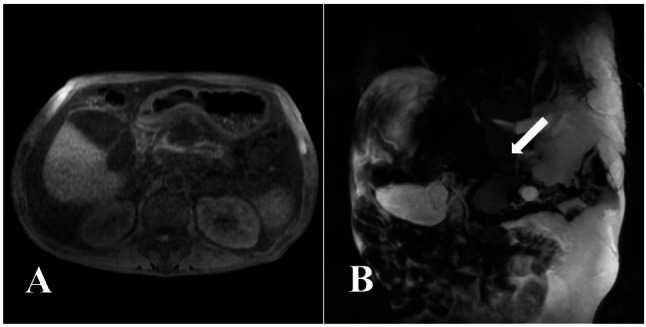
(A) MRI T1-weighted imaging sequence reveals an irregularly shaped cystic fluid signal in the pancreatic body, accompanied by volume loss and heterogeneous signal intensity in the body and tail. (B) Coronal MRCP demonstrates a tubular fluid-signal tract (white arrow) extending superiorly from the pancreatic body through the esophageal hiatus into the left pleural cavity
